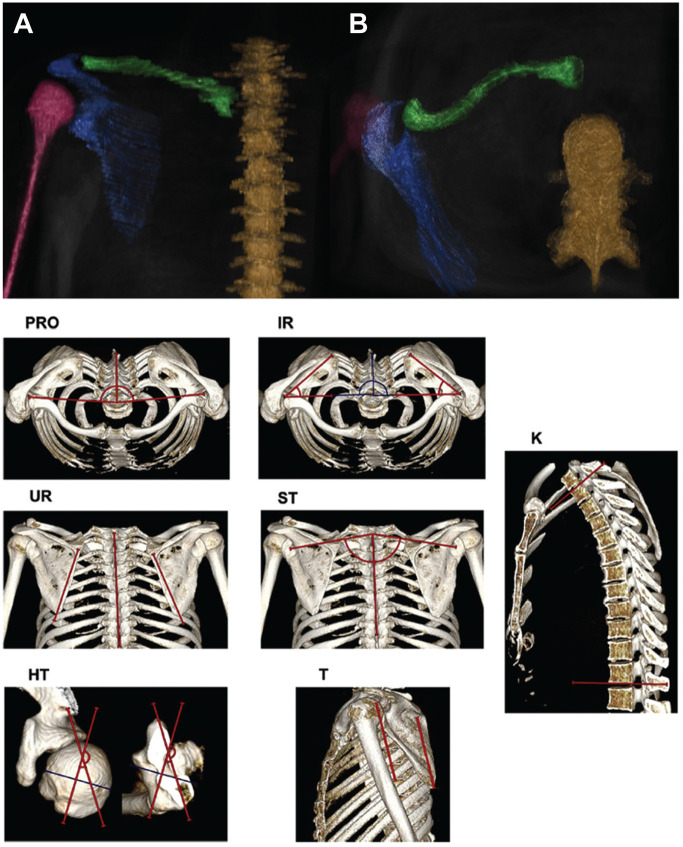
Three-dimensional (3D) models—(A) posterior and (B) axial views—were rendered from magnetic resonance imaging data in patients with C1 posterior shoulder instability to measure scapulothoracic orientation: spine (brown), clavicle (green), scapula (blue), and humerus (purple). Example measurements on 3D computed tomography models for scapular protraction (PRO), scapular internal rotation (IR), scapular upward rotation (UR), scapular translation (ST), global thoracic kyphosis (K), humeral torsion (HT), and scapular tilt (T) from the study of Moroder et al.^
